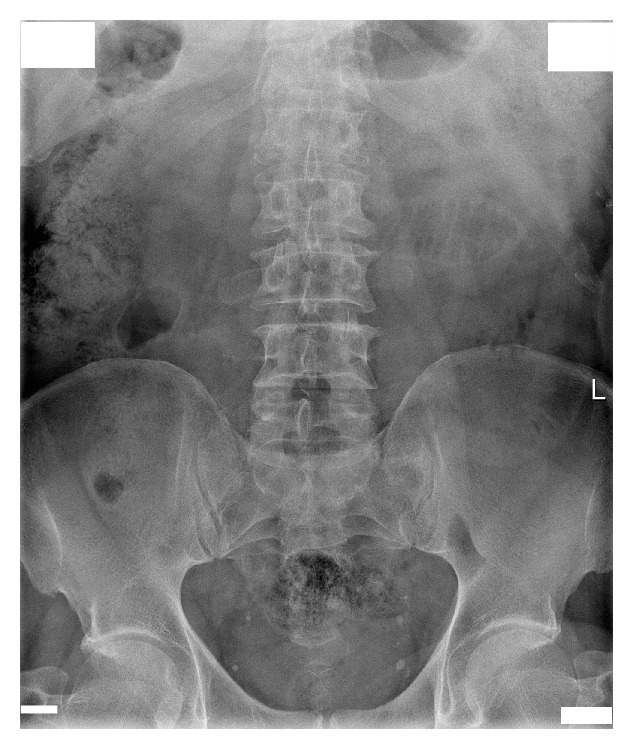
Plain X-ray image shows complete removal of the nitinol mesh stent and incrustations after percutaneous nephrolithotomy.
